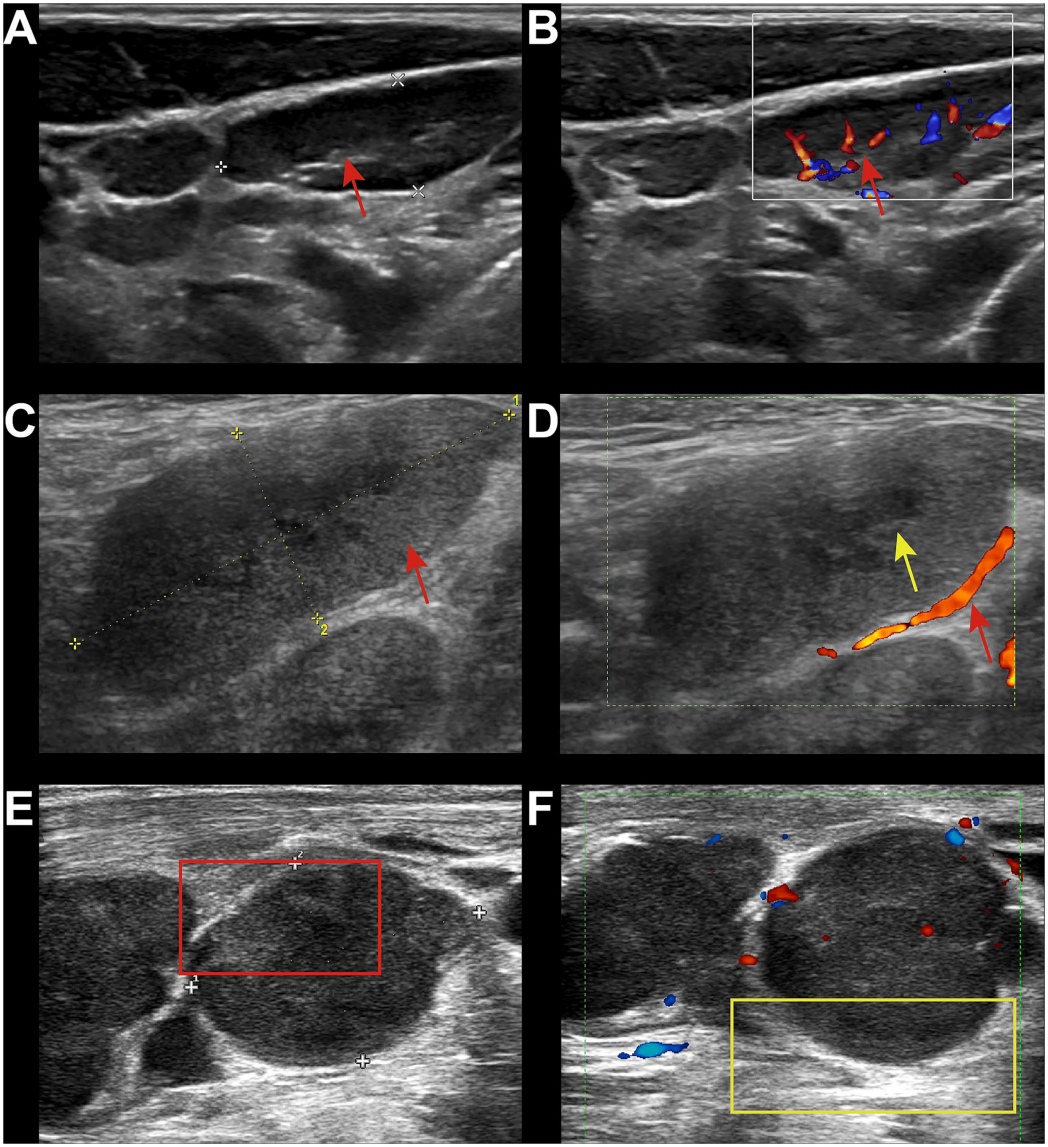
Ultrasound images of benign group. (A) 24-year-old male, diagnosed with HIV infection for 4 years. Left cervical lymph node showed enlargement with regular shape, clear margins, and visible lymph node hilum (red arrow). (B) CDFI showed abundant hilar blood flow (red arrow). The pathological diagnosis was reactive lymphoid hyperplasia. (C) 36-year-old male, diagnosed with HIV infection for 6 years. Multiple lymph node enlargements in the right axilla with regular shape had increased internal echogenicity (red arrow). (D) A few cystic changes were visible (yellow arrow), with the absence of the lymph node hilum. CDFI showed minimal peripheral blood flow (red arrow). The pathological diagnosis was fungal infection, with the final culture result identifying Talaromyces marneffei infection. (E) 48-year-old male, diagnosed with HIV infection for 8 months. Multiple lymph node enlargements were in the right neck with regular shape and heterogeneous internal echogenicity (red box). (F) The lymph node hilum was absent, and surrounding soft tissue edema was present (yellow box). CDFI showed minimal peripheral blood flow signals. The pathological diagnosis was TB.
